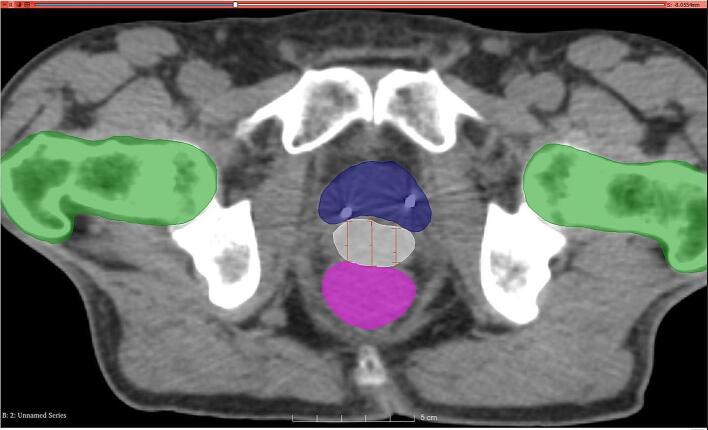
CT scan of balloon spacer between the rectum and prostate in axial view. Spacer measured at midline, to that 1 cm bilaterally from midline.
